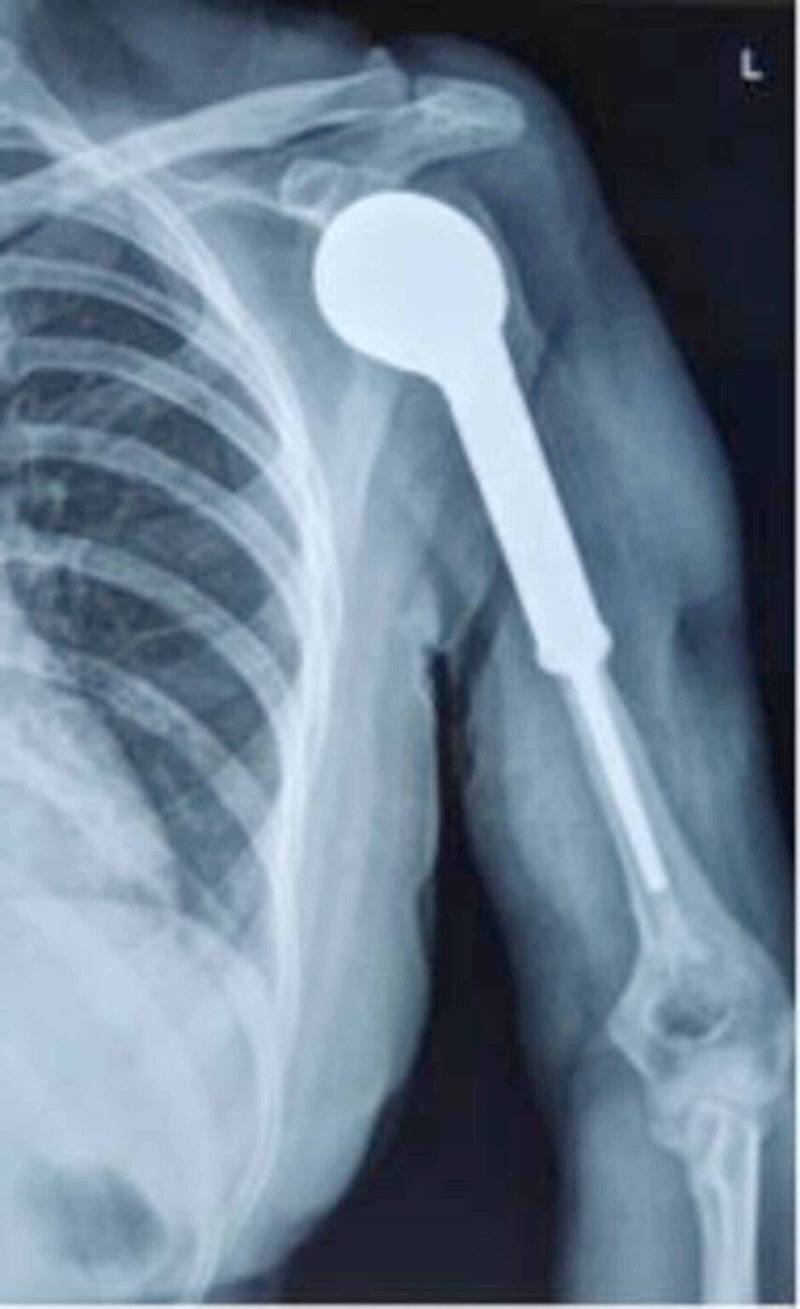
Postoperative follow-up X-ray in 2020 showing Restor Mega Prosthesis for the proximal humerus in position and with good fixation
